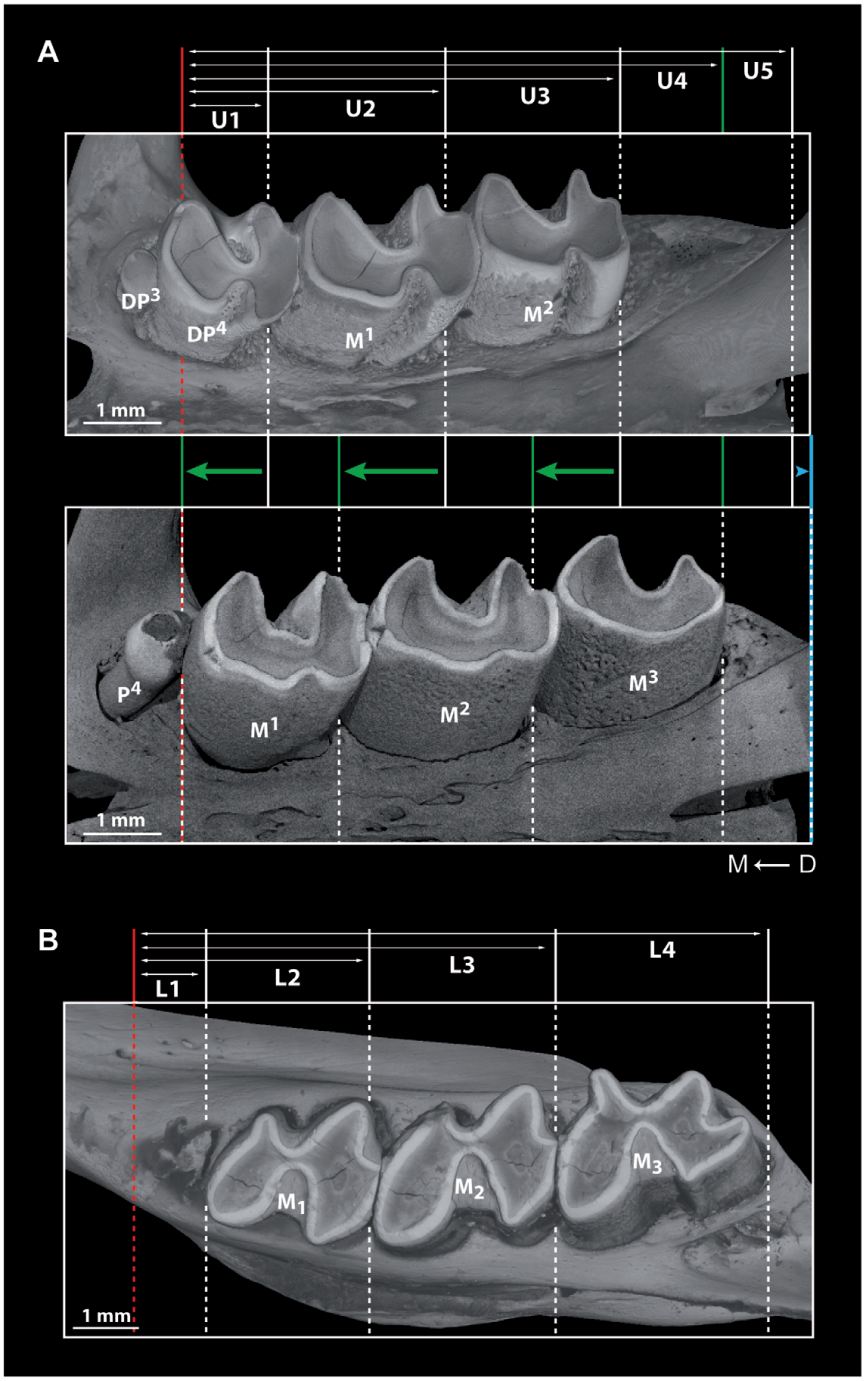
Characterization of dental mesial drift by using skull measurements (see material and methods for details). A, X-ray synchrotron and conventional microtomographic 3D rendering of left upper cheek tooth rows of *Pectinator spekei* (MNHN-CG1895-461 and 1995-19); green arrows indicate dental drift, blue arrow indicates maxillary growth. B, X-ray synchrotron microtomographic 3D rendering of left lower cheek tooth row of *Felovia vae* (MNHN-CG 1989-22). D → M stands for distal to mesial direction.
